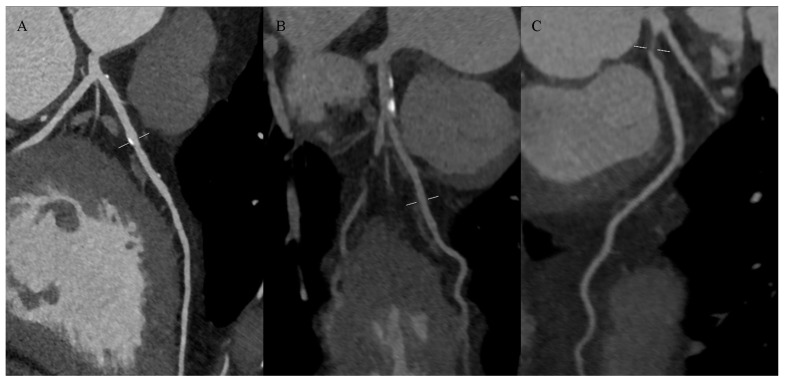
Atherosclerotic plaques on coronary computed tomography angiography: (A) calcified plaques in the left anterior descending artery (LAD), (B) mixed plaques in the LAD, (C) non-calcified concentric plaque in the LAD.
